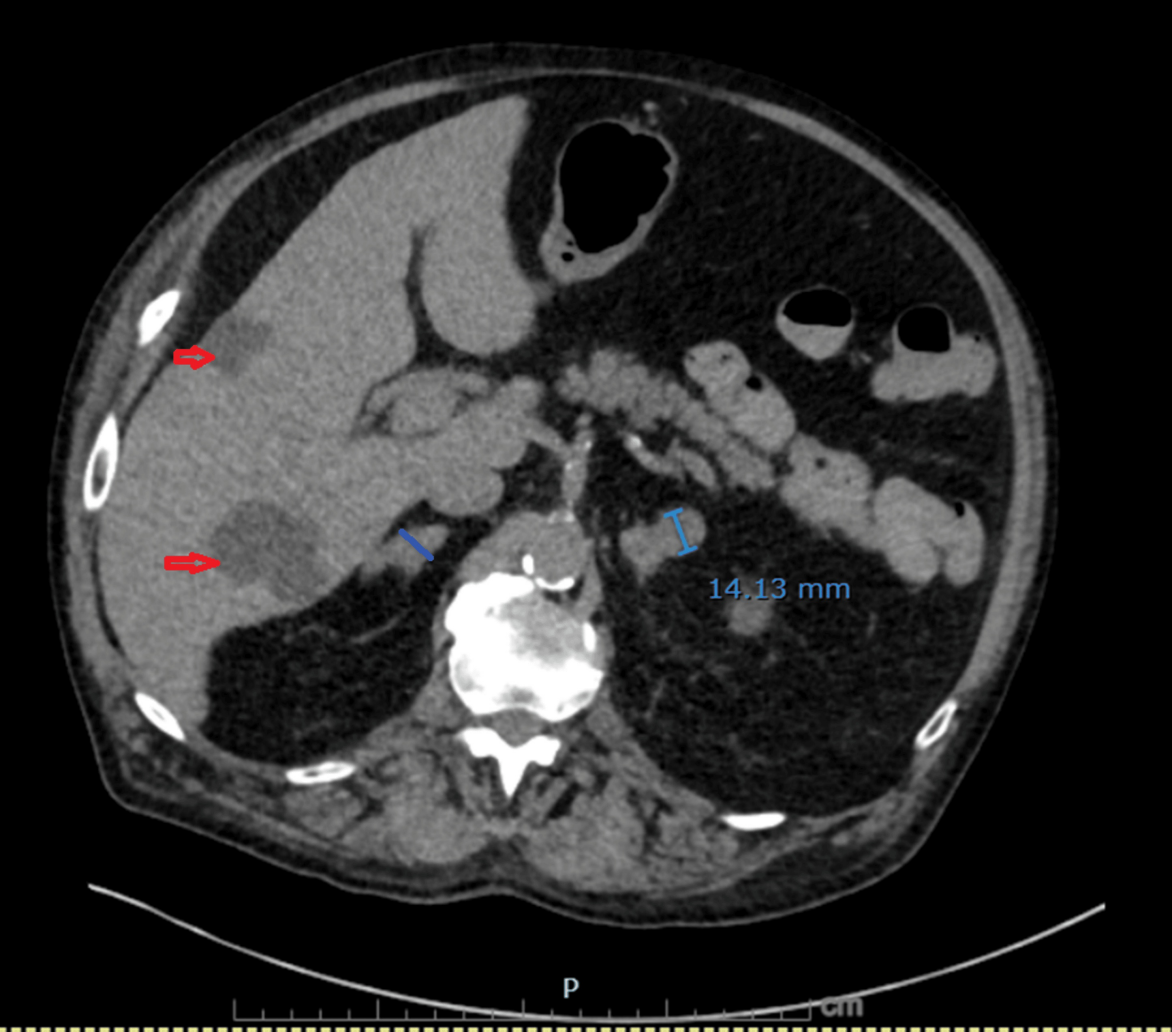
Non-contrast CT demonstrating bilateral adrenal metastases Nodular enlargement of both adrenal glands has progressed compared with prior imaging: the left adrenal lateral limb measures 14 mm (previously 9.2 mm) and the right adrenal body measures 12.4 mm (previously 7 mm). Multiple benign hepatic cysts are also visualized (red arrows).
